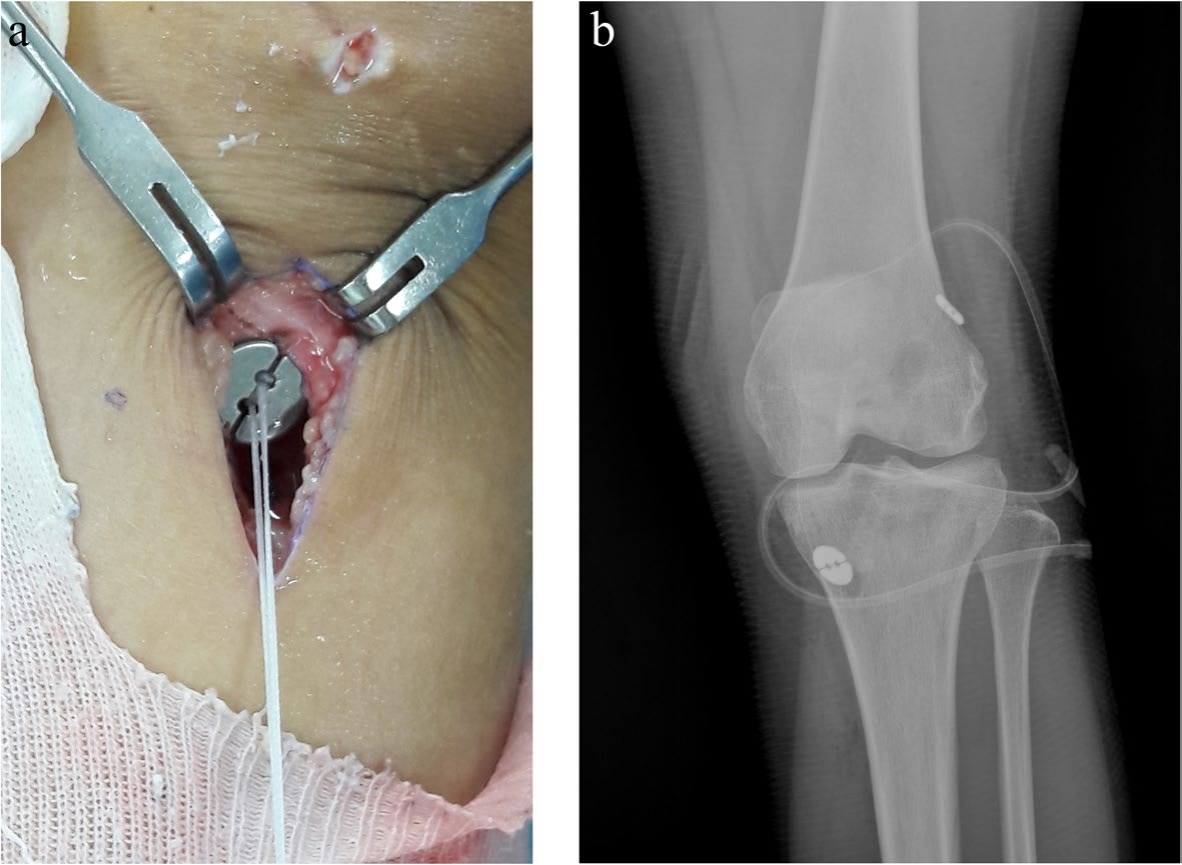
a Modified hybrid fixation with TightRope ABS implants (Arthrex) on the tibial side. b Postoperative radiograph revealing application of suspensory devices in modified hybrid tibial fixation
